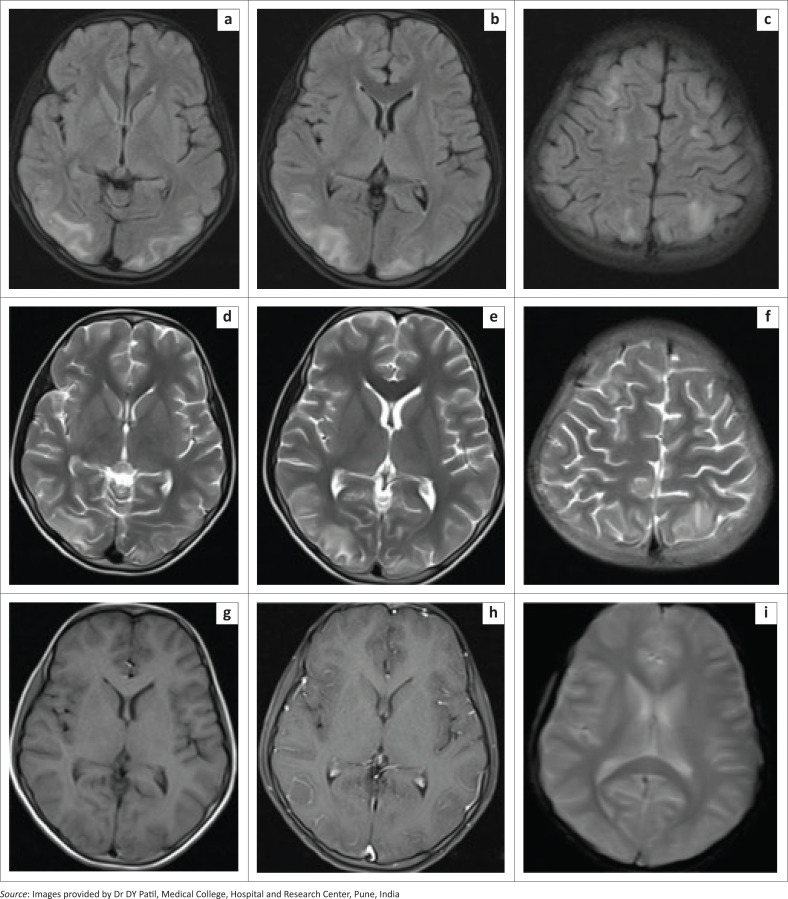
[Case 5]: Brain MRI of a 10-year-old female with NS1 antigen seropositive status showing multiple symmetrical patchy areas of FLAIR (a-c) and T2-weighted (d-f) hyperintensities in the bilateral temporo-occipital regions and to a lesser extent in the frontoparietal regions, involving the cortex and subcortical white matter. These areas showed T1 iso to hypointense signal (g) with no post-contrast enhancement (h) and no blooming on GRE images (i).
